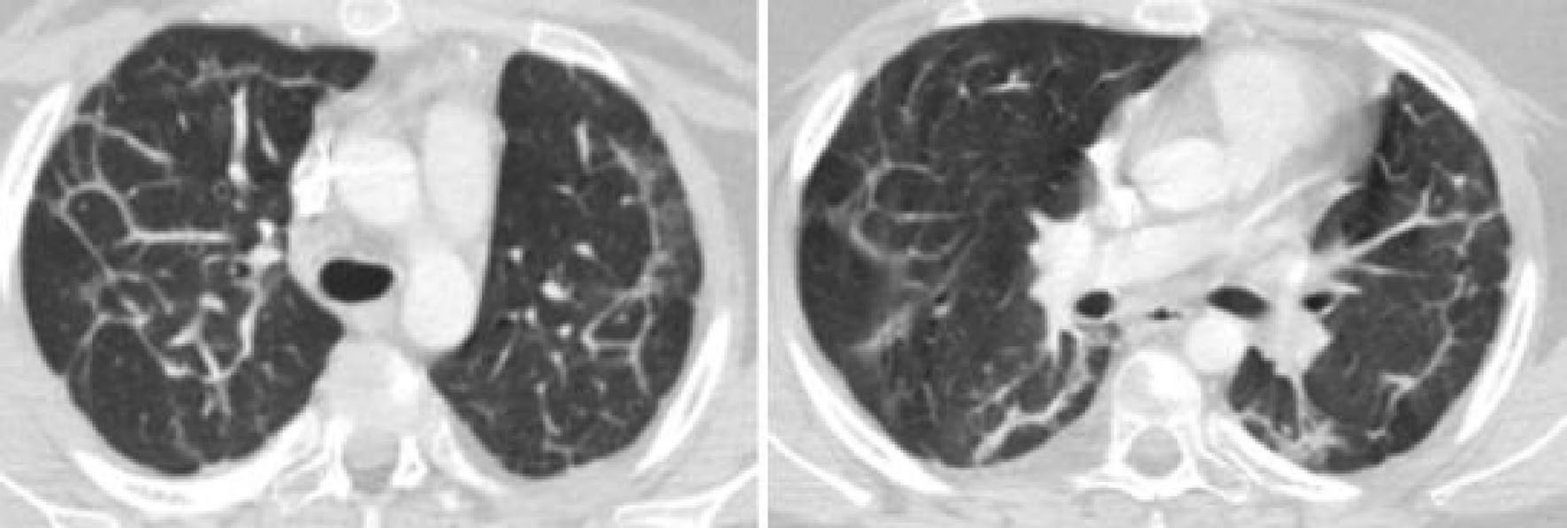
Fibrotic changes decreased with treatment on thorax CT
